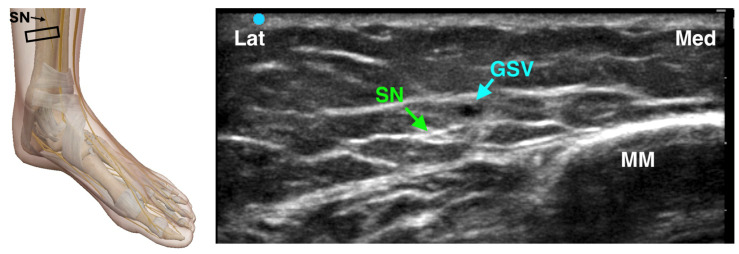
Ultrasound-guided Saphenous Nerve Block. SN: Saphenous Nerve; GSV: Great Saphenous Nerve; MM: Medial Malleolus; The black rectangle indicates the placement of the ultrasound probe on the anatomical area.
